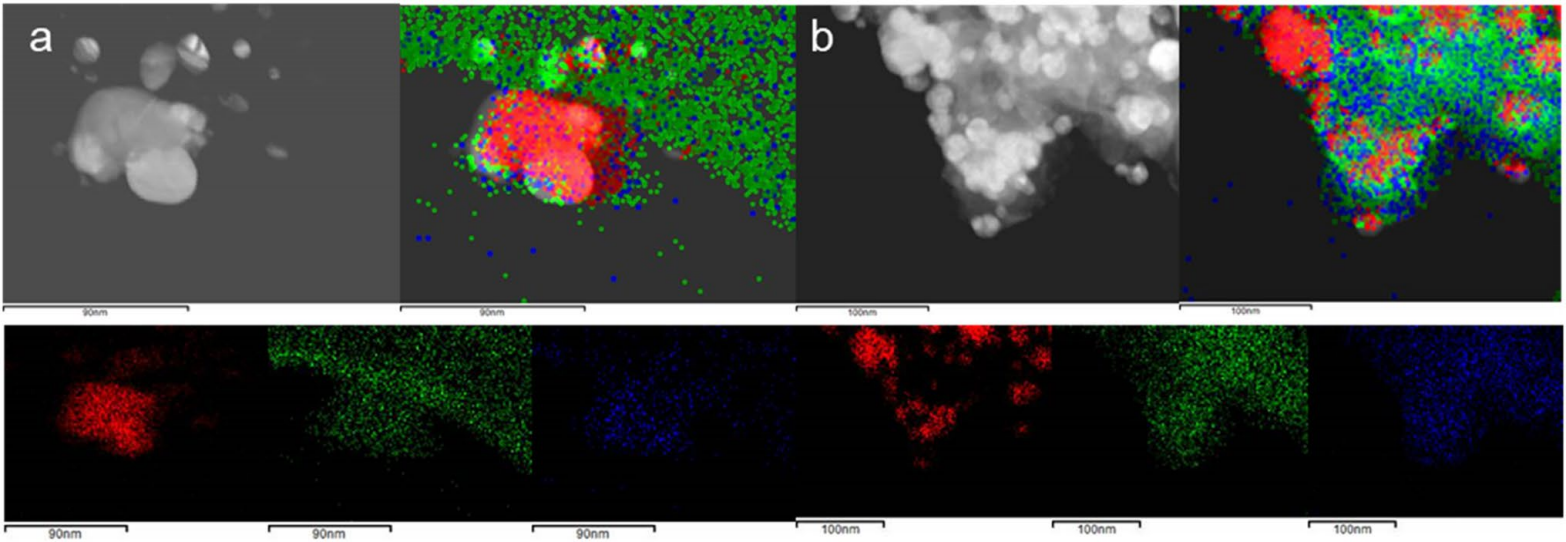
STEM images and elemental mappings [silver (red), carbon (green) and oxygen (blue)] of a Ag@CB and b Ag@CT
